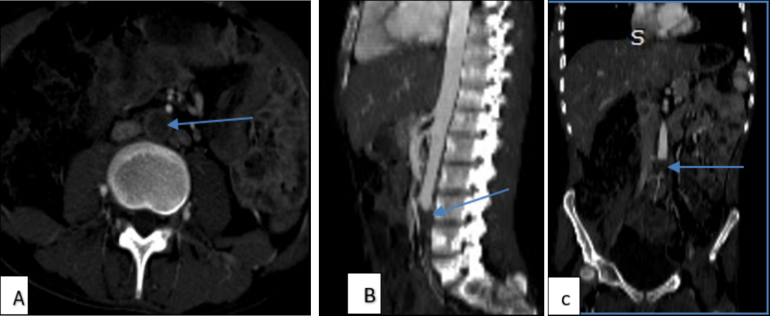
A) Axial, B) sagittal, and C) coronal views of abdominal CT angiography show a hypodense filling defect located in the distal abdominal aorta infra renal at the L3 level and absence of flow below the defect.
